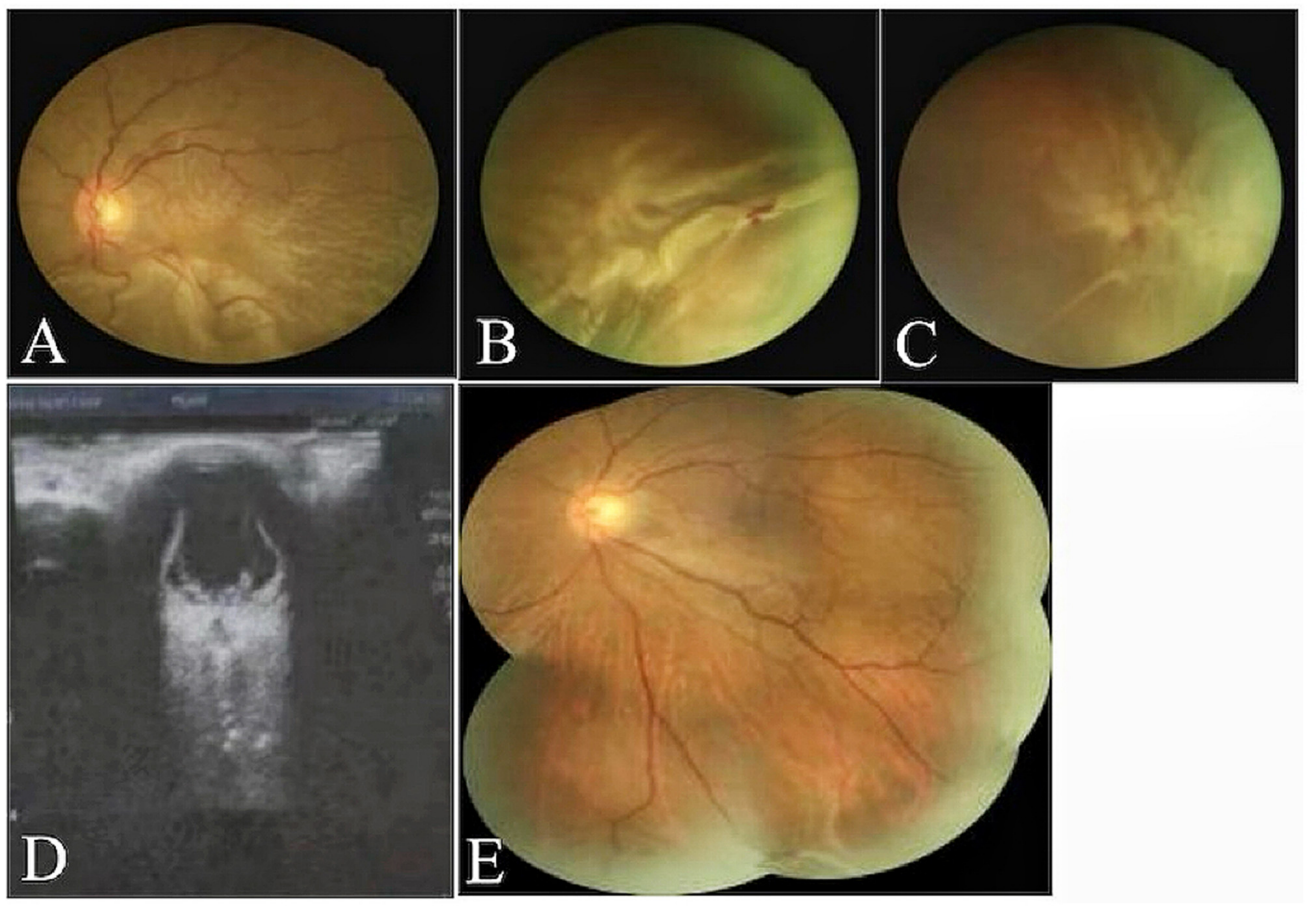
(A–D) Fundus photography and ocular ultrasound demonstrated pre-operative total retinal detachment and proliferative retinal changes in the patient. (E) One month post-operative complete retinal reattachment in patients undergoing combined SB and pure C3F8 gas filling.
